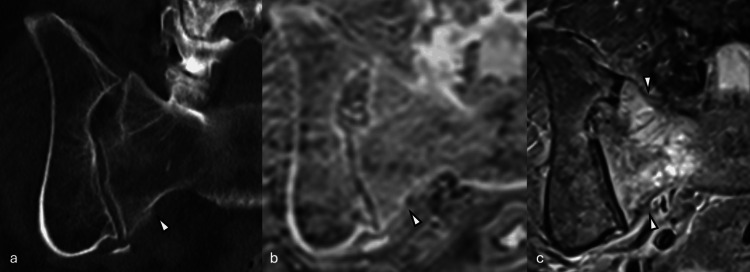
Representative imaging example 4. A patient with a minimally displaced right sacral ala fracture (arrowheads) on (a) coronal CT, (b) coronal pseudo-CT and (c) coronal short tau inversion recovery MR. This case was correctly identified by one of the readers on pseudo-CT but missed by both readers in CT.
